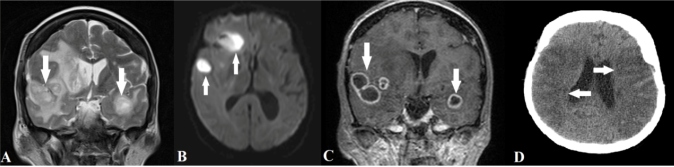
T2-weighted (A), diffusion-weighted (B), and gadolinium-enhanced T1-weighted magnetic resonance images (C) showing multiple hyperintense lesions, with restricted diffusibility and peripheral enhancement affecting the bilateral temporal and right frontal lobes (white arrows), suggesting abscesses; brain computed tomography (D) revealing an ischemic vascular insult in the territory of the middle cerebral arteries (white arrows).
